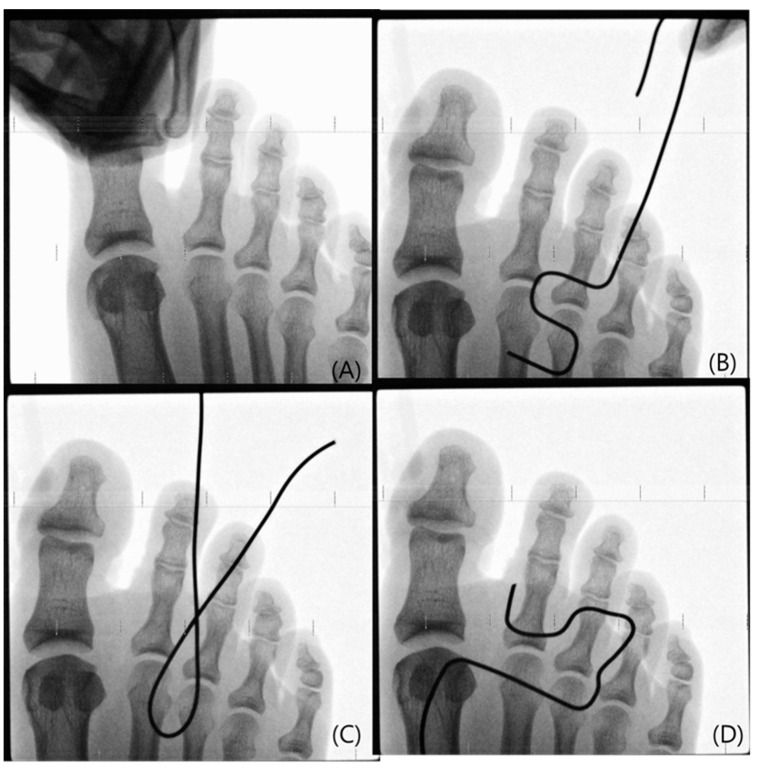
Anterior–posterior foot plain radiographs taken using a mini-fluoroscan with manual traction: (A), 5 lb (B), 10 lb (C), and 15 lb (D) of traction. These plain radiographs were computerized and used to measure the MTP joint space with a PACS system. The lines in these plain radiographs are wire lines representing the distraction pounds.
